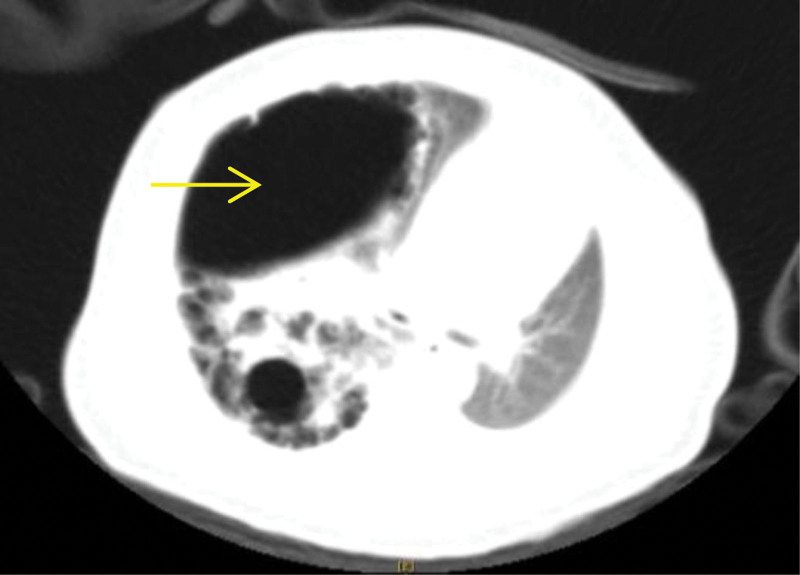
CT at 2 days after birth suggested cystic lesions of variable size in the right lung, mediastinum shift, and compression of the left lung and heart. CT = computed tomography.
